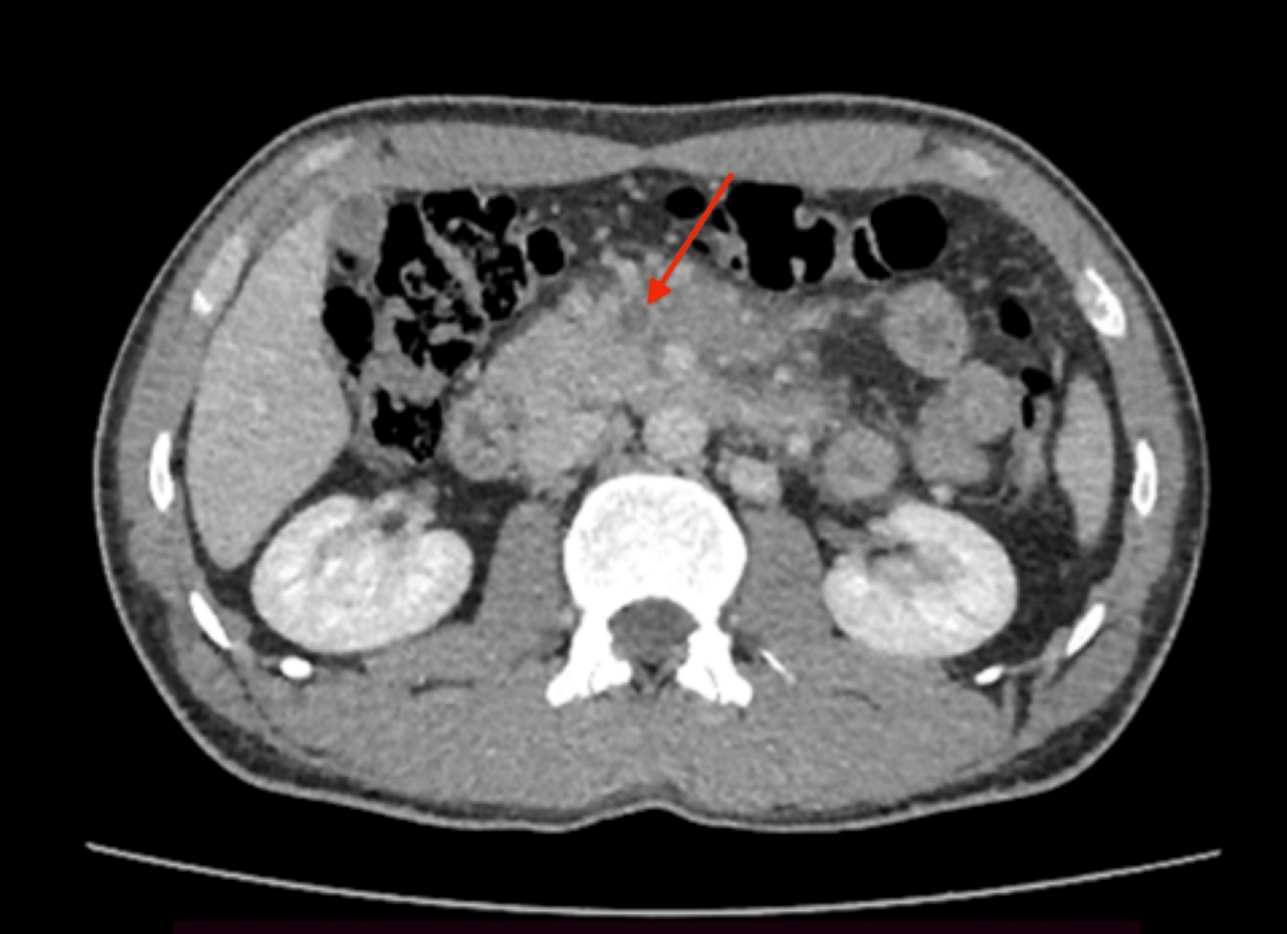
Axial abdominal CECT in the portal venous phase showing a filling defect in the superior mesenteric vein (arrow) CECT: contrast-enhanced computed tomography.
